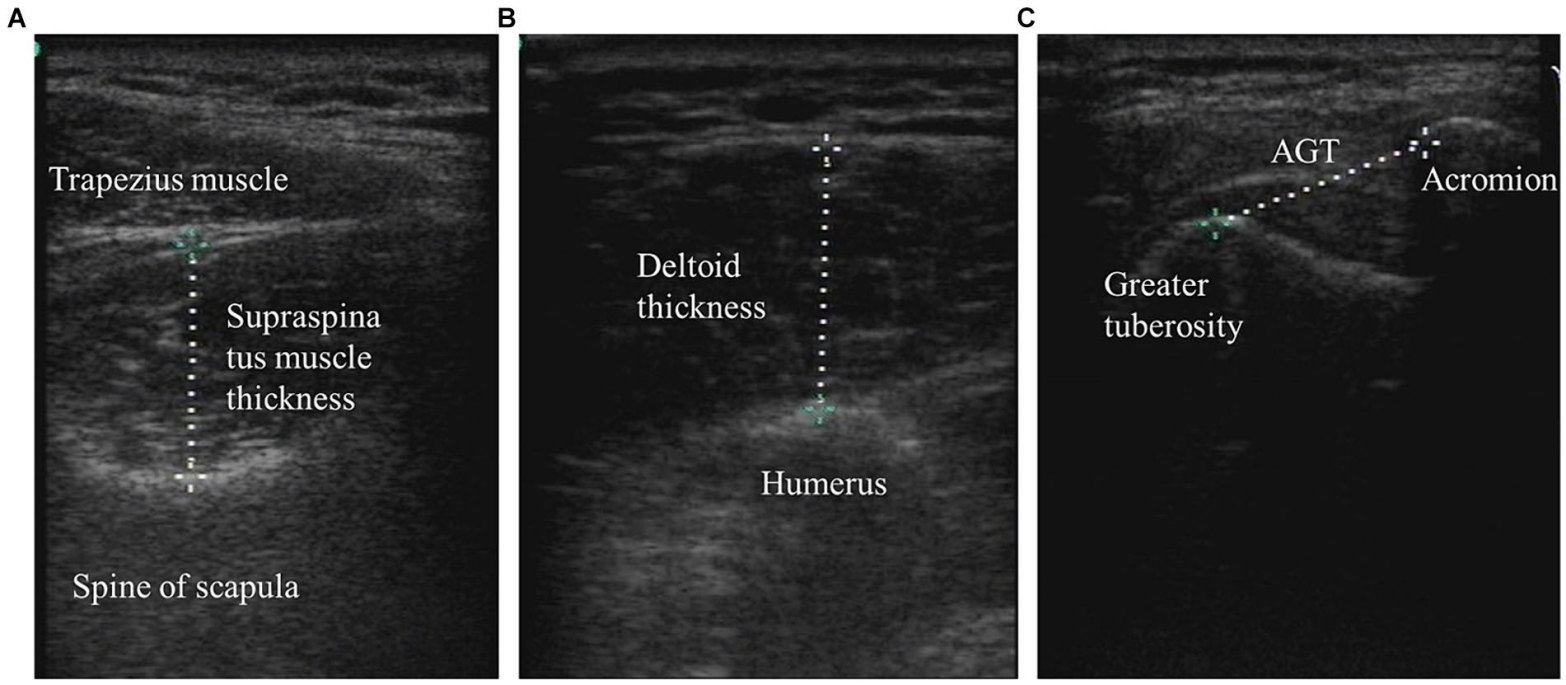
The supraspinatus muscle thickness, deltoid muscle thickness, and AGT were measured by ultrasound imaging. (A) Supraspinatus muscle thickness. (B) Deltoid thickness; and (C) Acromion-greater tuberosity distance.
